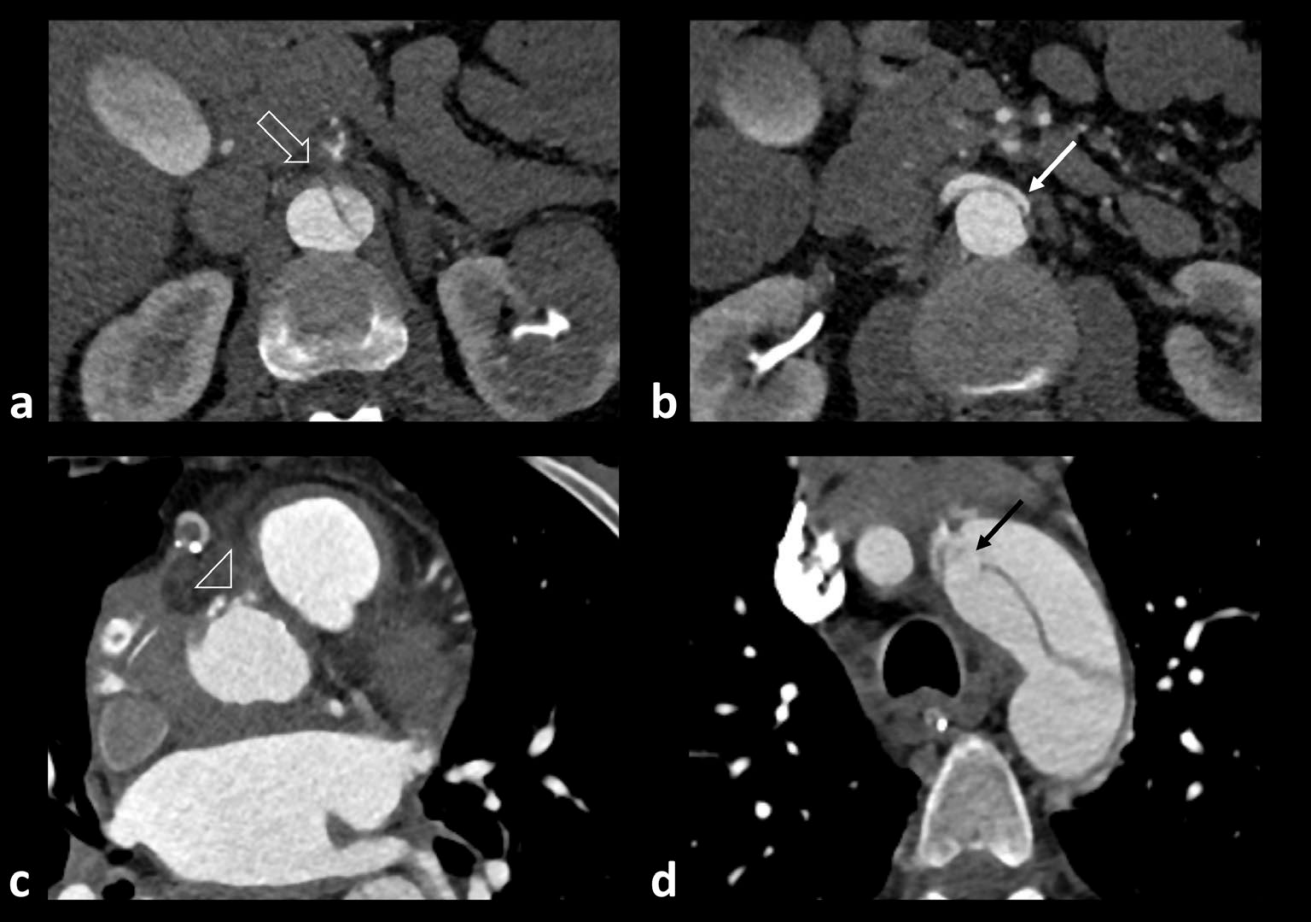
Postoperative findings in type‐A dissection with right coronary compression and aortic re‐entry. (a) Axial abdominal computed tomography angiography (CT‐A) after surgical treatment indicated compression of the right coronary artery by absorbable hemostyptic agent (white arrowhead). (b, c) Furthermore, it revealed a wide re‐entry of the aortic arch (black arrow) and a small re‐entry infrarenal (white arrow). (d) The chronic occlusion of the celiac trunk (white bold arrow) which was previously compensated via gastroduodenal collaterals but is currently accompanied by distinct hepatic malperfusion (not shown).
